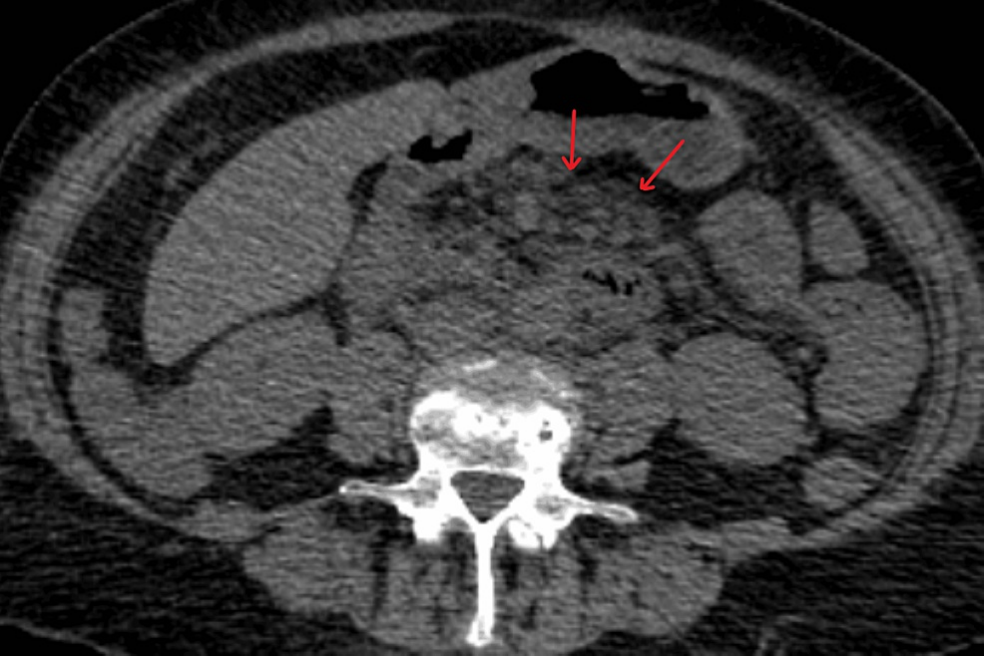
Non-contrast axial CT abdomen slice at the level of the root of mesentery suggestive of mesenteric panniculitis The red arrows point to an area of fat stranding and nodularity indicating focal extensive inflammatory changes around the root of mesentery
